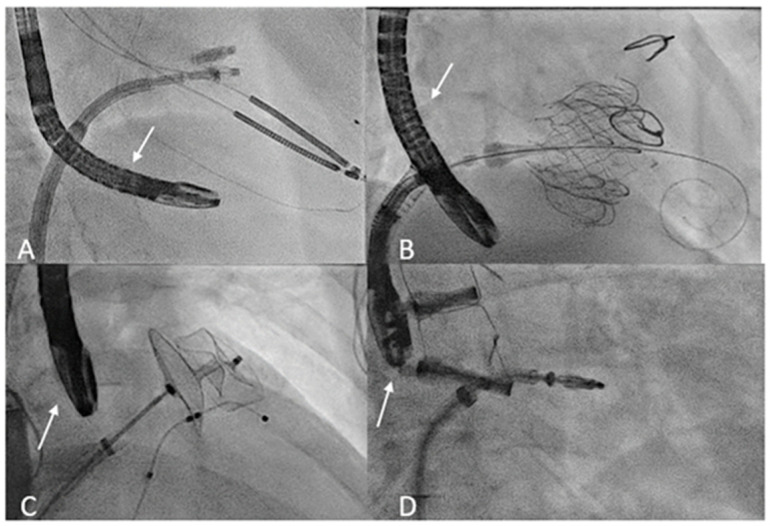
Transoesophageal probe position (arrow) during transcatheter interventions. (A) The fluoroscopy shows the transgastric probe position during T-TEER. (B) Deep esophageal view used during tricuspid valve replacement. (C) Mid esophageal probe position during LAA occlusion. (D) Mid esophageal probe position used during M-TEER. T-TEER: Tricuspid Transcatheter edge-to-edge repair; LAA: left atrial appendage; M-TEER: Mitral Transcatheter edge-to-edge repair.
